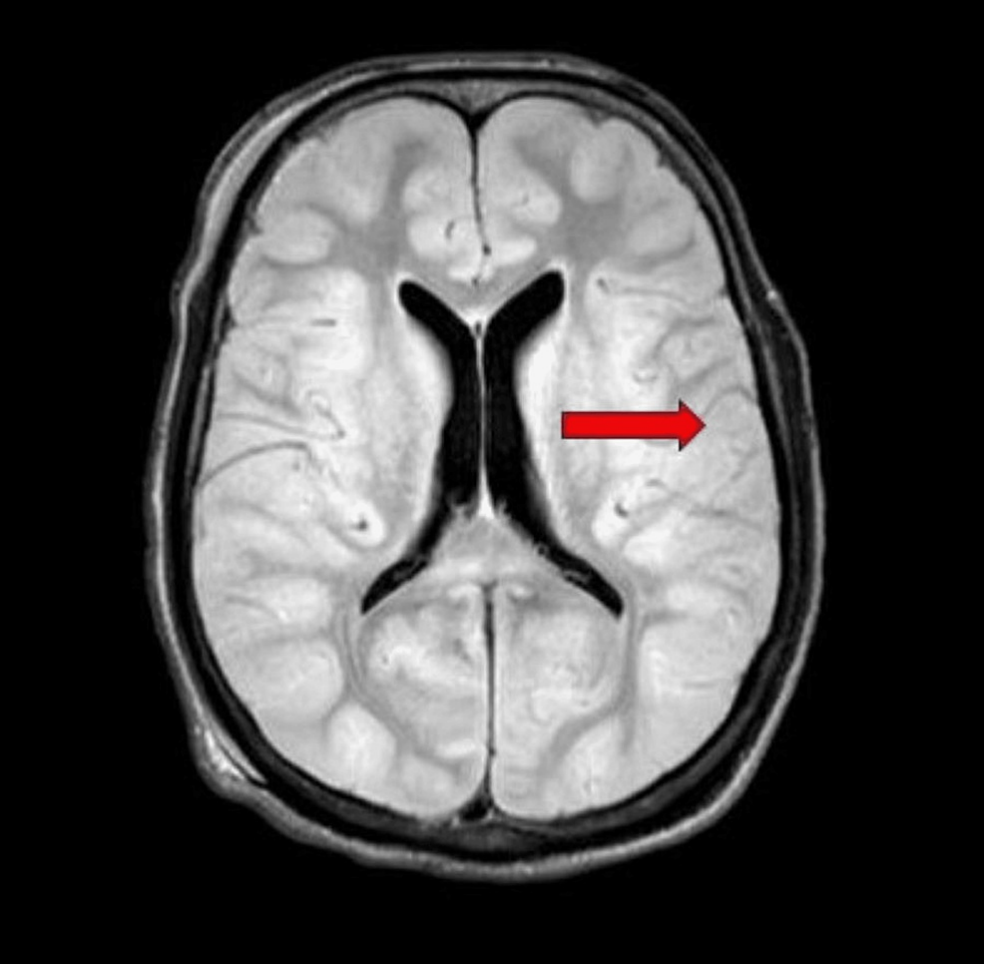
Magnetic resonance image of the brain of the patient in FLAIR sequence showing diffuse cerebral edema (red arrow). FLAIR: fluid-attenuated inversion recovery
